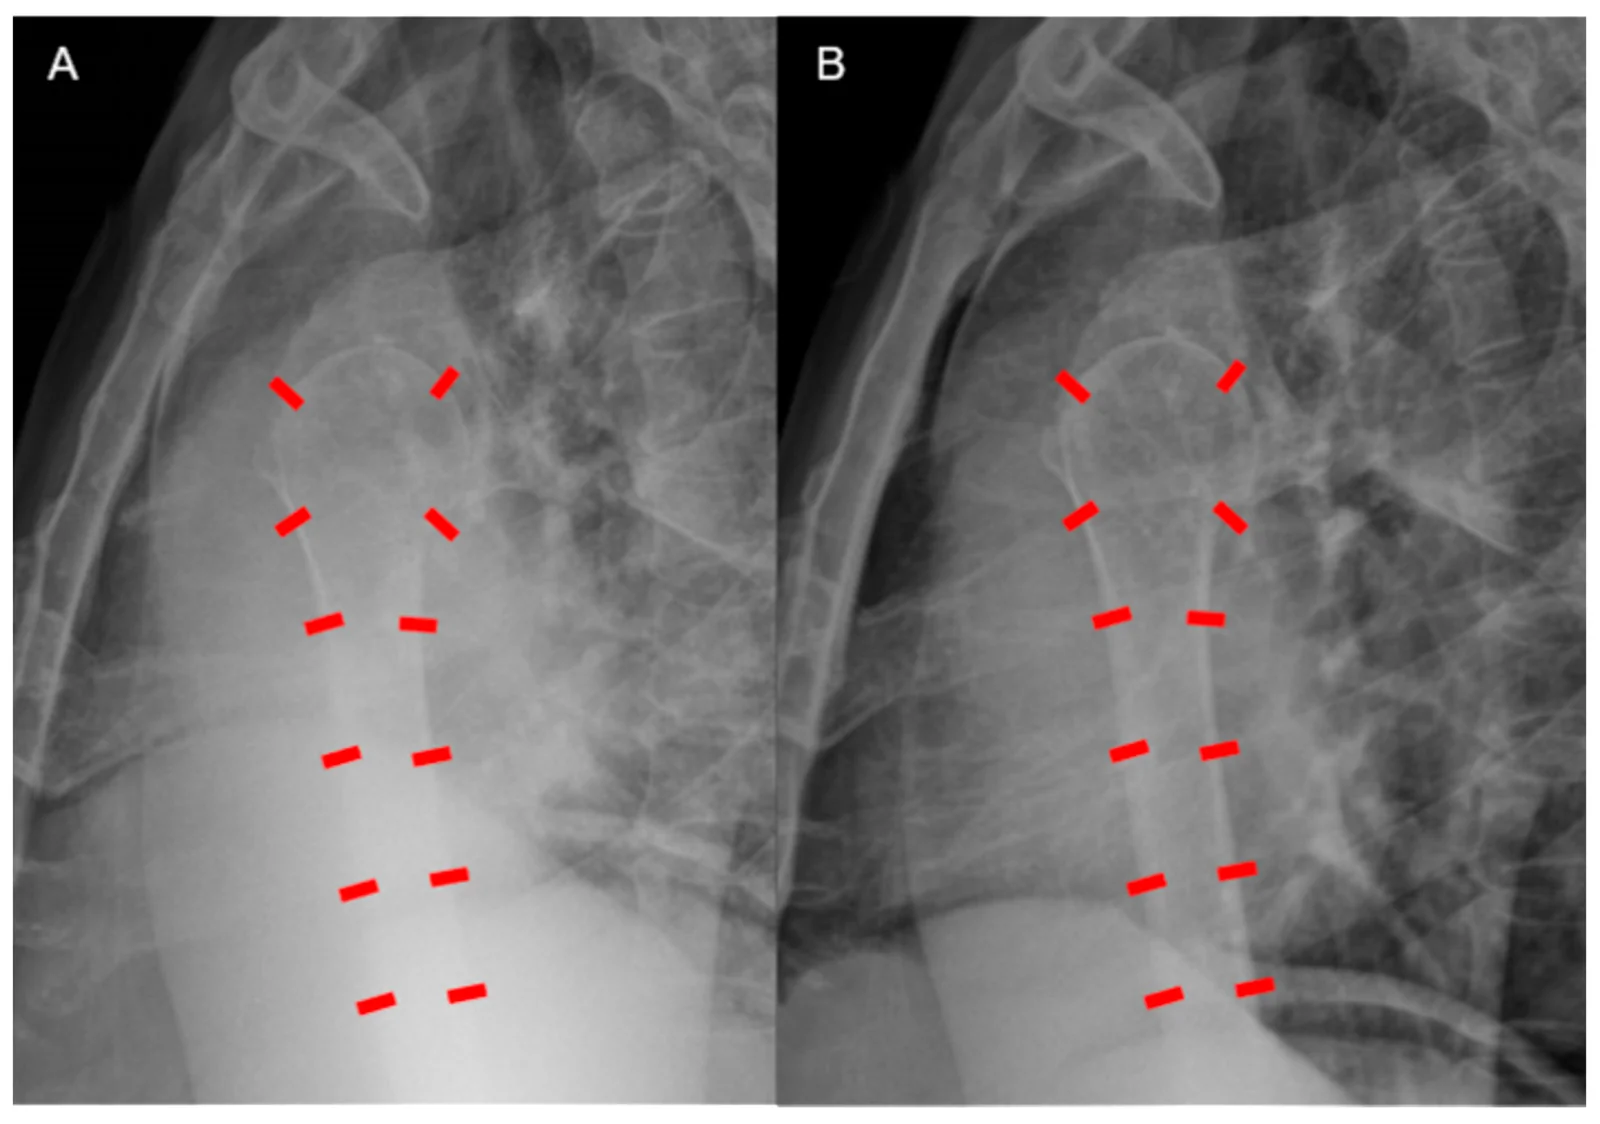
The placement of short lines for measuring edge sharpness of the humerus and adjacent tissues using ImageJ software on free-breathing (A) and deep-inspiration breath-hold (B) transthoracic lateral shoulder radiographs.
